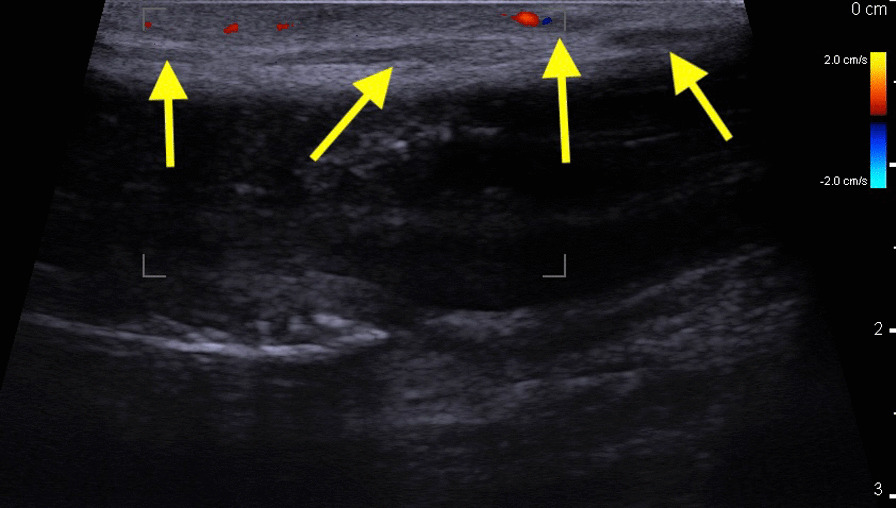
Doppler ultrasound of penis: longitudinal section. Yellow Arrow—Dorsal Superficial penile vein without flow
